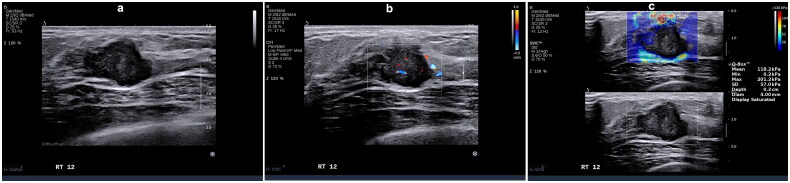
A 37-year-old female with right breast invasive ductal carcinoma grade II (score of 7), tumor size 2.0 × 1.8 × 1.5 cm, luminal B (HER2+) subtype. (a) Ultrasonography features: irregular shape, angular and lobulated margins, enhanced posterior echo, multiple punctate calcifications. (b) Adler blood flow grading – grade 2. (c) Emax = 201.2 Kpa.
